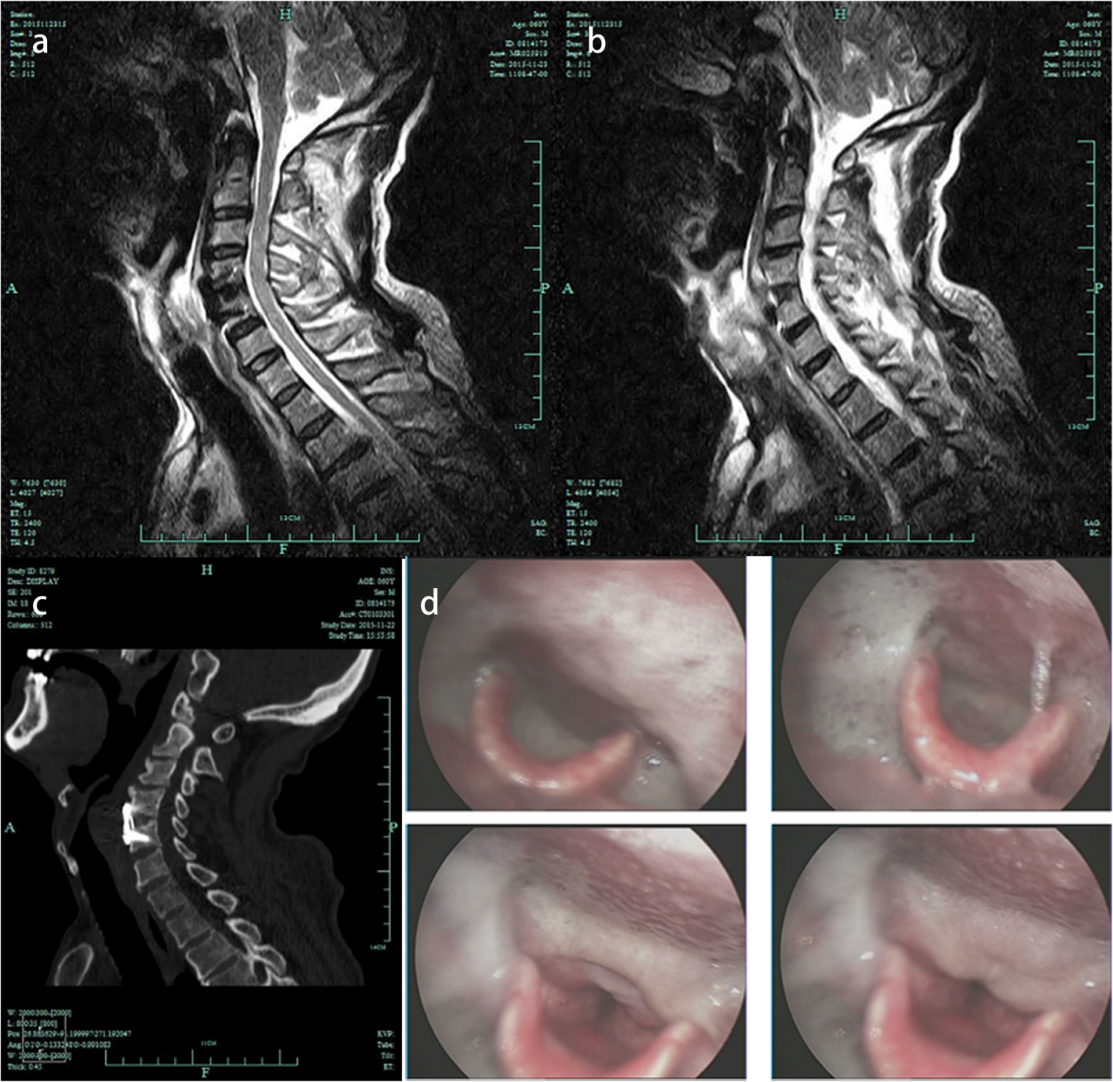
Imaging done five months after ACDF. a, b T2-weighted sagittal image demonstrated extensive high signal in throat tissue at the C4–5 level. c Computer tomography showed that the plate and cage were in their original positions without looseness or displacement. d Laryngoscope revealed edema and whiteness in the throat tissue without obstruction
